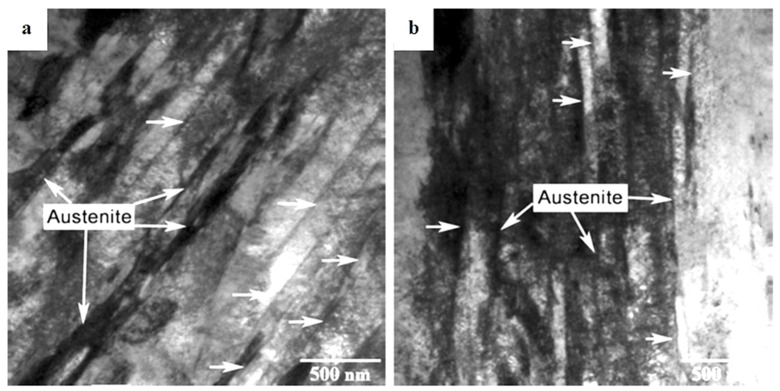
TEM microstructures of retained austenite in 0.15% C, 14% Cr, 12.5% Co, 4.5% Mo, and 2% Ni steel after different heat treatment processes. (a) Austenitisation at 1050 °C for 40 min, followed by oil quenching; (b) austenitisation at 1050 °C for 40 min, followed by oil quenching and CT at −196 °C for 2 h. Adapted from [70].
